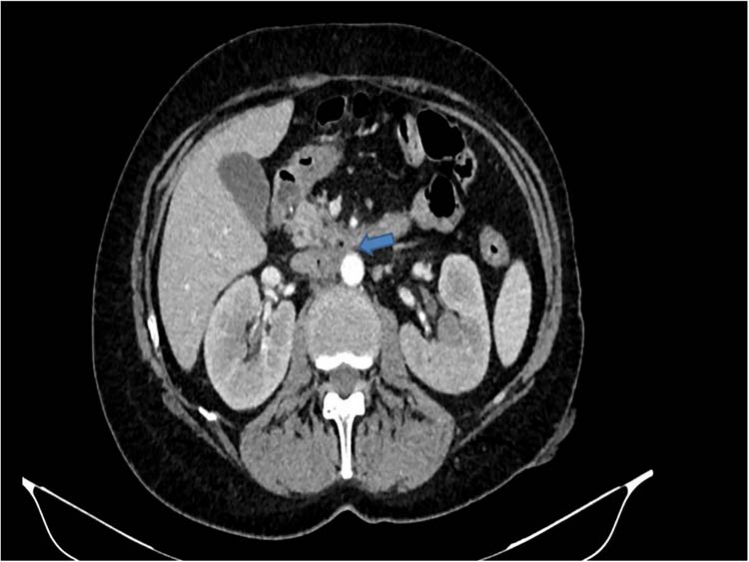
CT scan showed the presence of strong adherence between the IVC at the origin of the left renal vein and the third duodenal portion.
